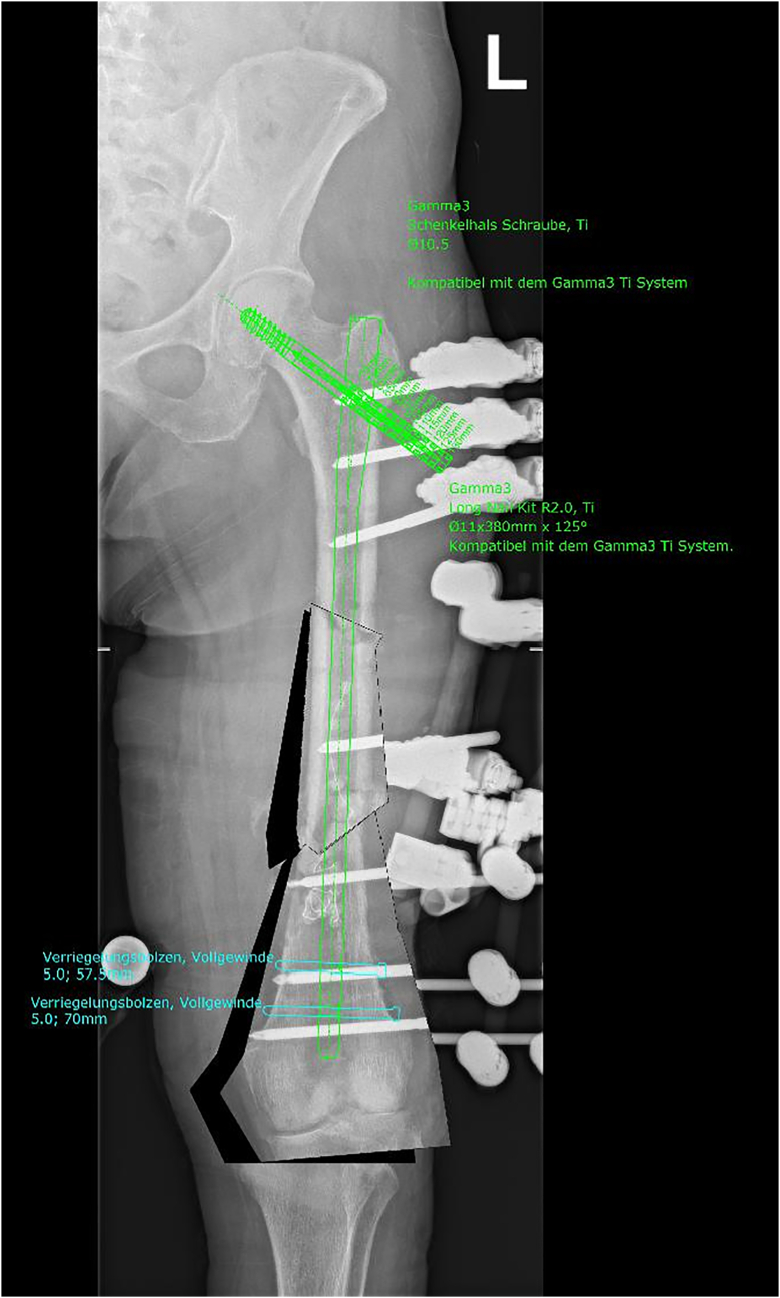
X-ray with software-based pre-operative planning with long gamma nail with cephalo-medullary screw and double distal locking.
